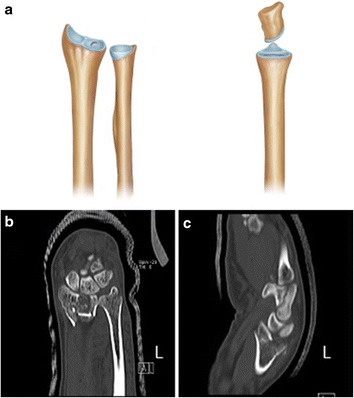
Center depression fracture. a The depression fracture occurs in the center of the lunate fossa of the distal radius (*left*, anterior view; *right*, lateral view). b, c Computed tomography (CT) (left)
